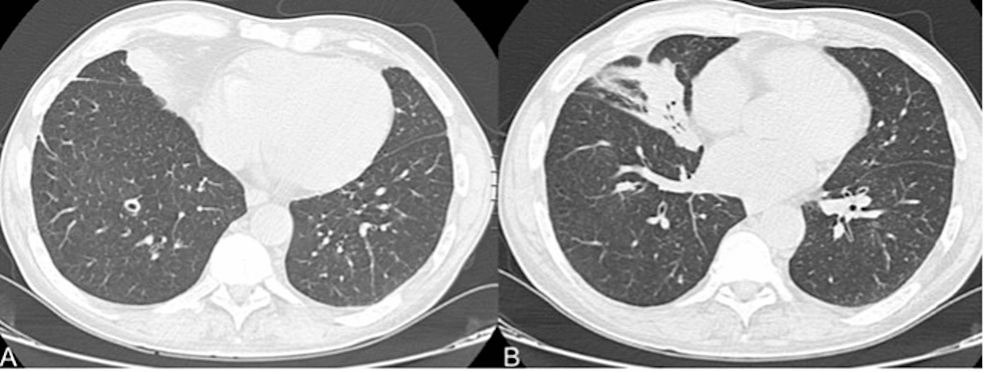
Pulmonary cavitary nodule on computed tomography in the lateral basal segment of the right lower lobe (A); parenchymal consolidation with aerial bronchogram in the medial segment of the middle lobe (B).
